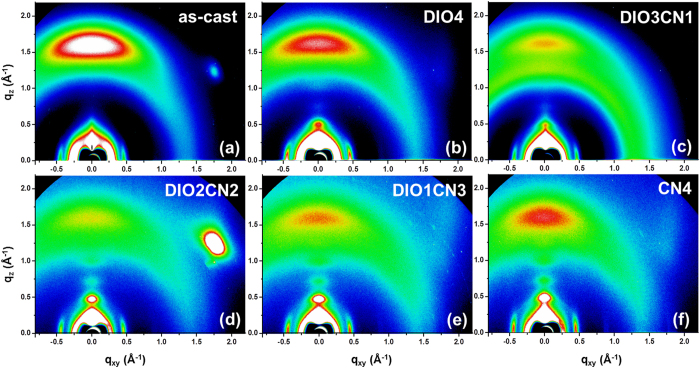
Two-dimensional GIWAXS images of the PBDTTT-CT:P(NDI2OD-T2) films with different additive treatments: (a) no additive, (b) DIO 4, (c) DIO/CN 3:1, (d) DIO/CN 2:2, (e) DIO/CN 1:3, and (f) CN 4.
